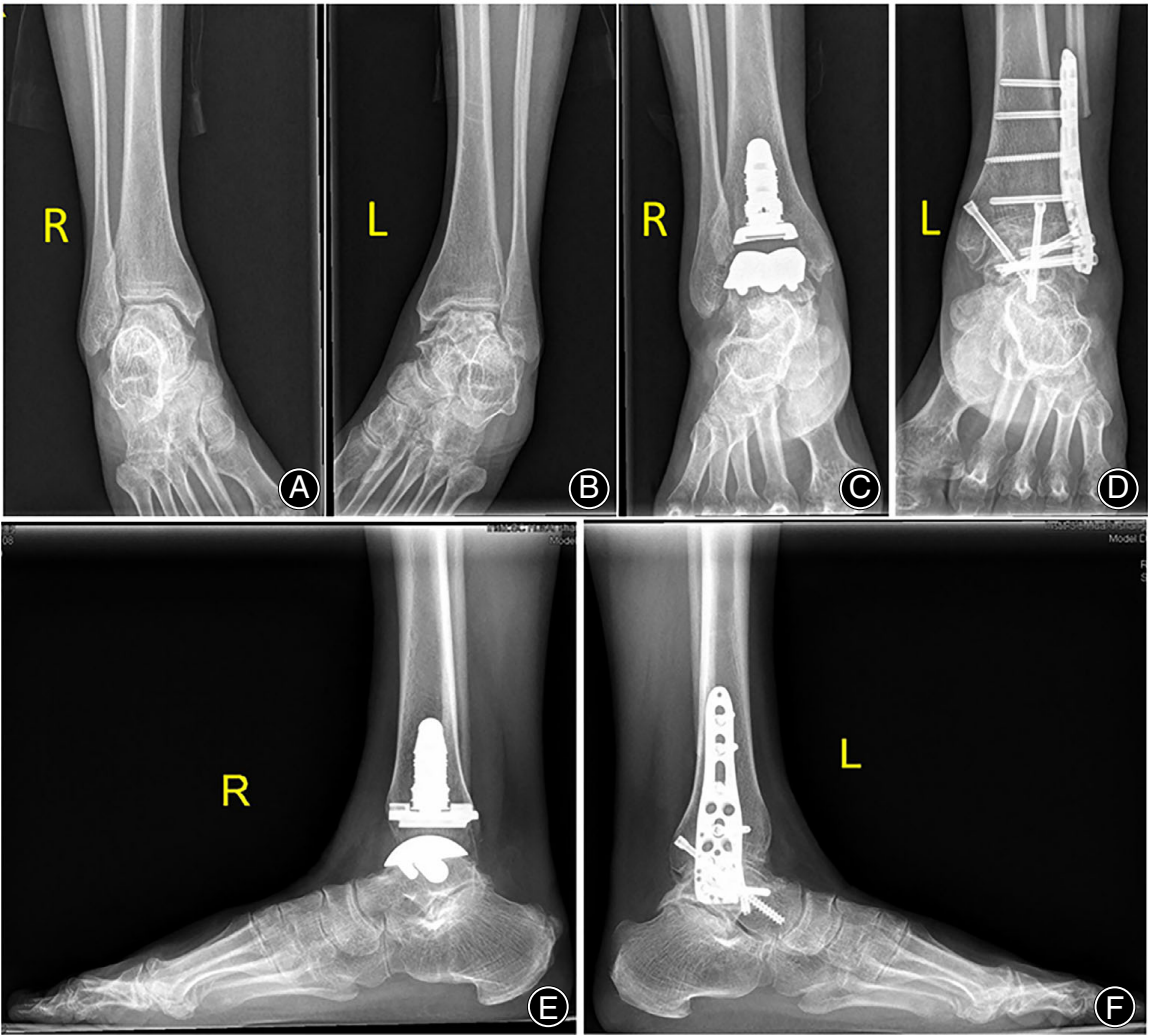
Reoperative and postoperative weightbearing radiographs of the arthroplasty and arthrodesis procedures. The patient was a 50‐year‐old female diagnosed with bilateral end‐stage ankle osteoarthritis (A, B). Anterior radiographs of INBONE II and arthrodesis (C, D). Lateral radiographs of INBONE II and arthrodesis (E, F)
